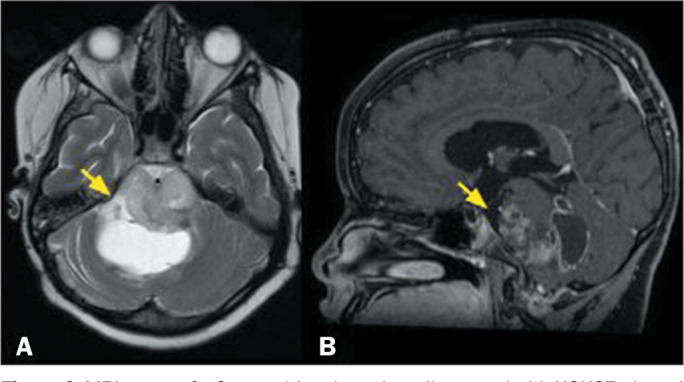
MRI scans of a 9-year-old male patient diagnosed with H3K27-altered
